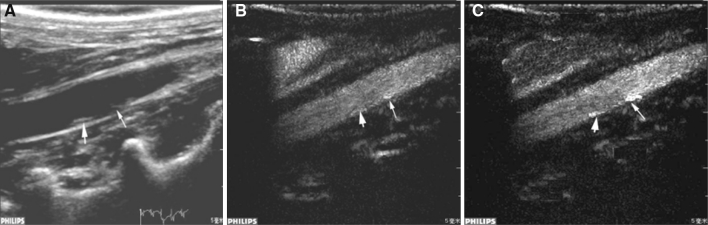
Representative ultrasound images of the atherosclerotic plaques in the rabbit abdominal aorta. a Two-dimensional image showing a plaque (*large arrow*); a soft plaque was invisible (*small arrow*). b Contrast ultrasound imaging with normal microbubbles showing two plaques with stronger echo compared with the two-dimensional view. The enhancement of the echo was more apparent for the soft plaque (*small arrow*). c Contrast ultrasound imaging with IL-8-targeted microbubbles showing two plaques with a markedly stronger echo compared with normal contrast ultrasound
